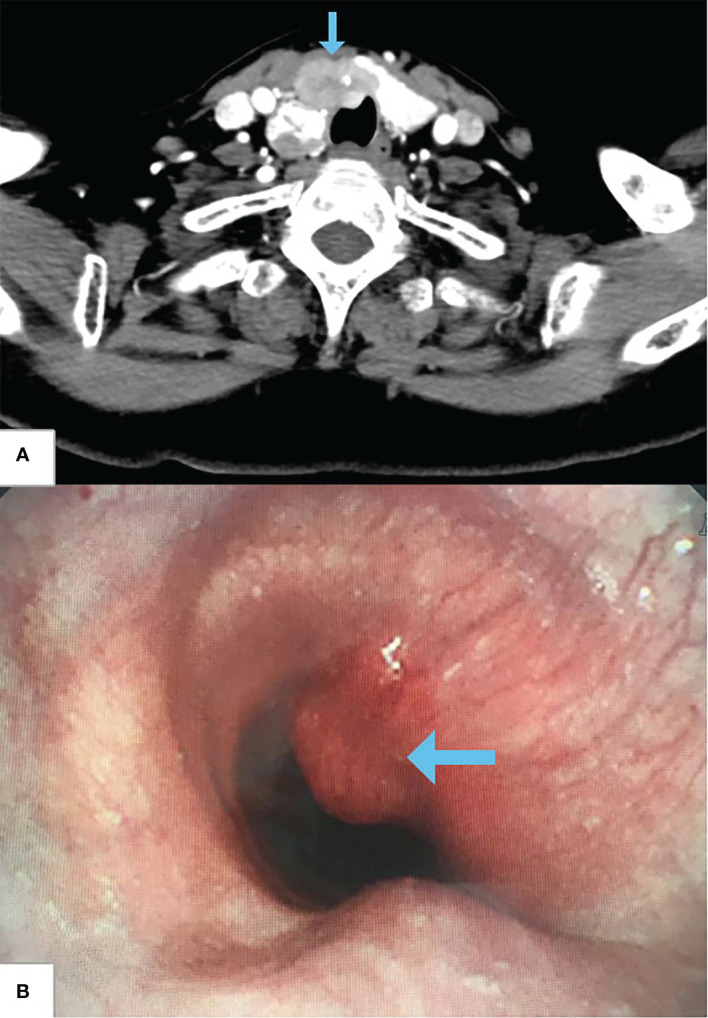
Preoperative cervical CT and fiberoptic bronchoscopy. (A) CT shows thyroid carcinoma invading trachea (arrow). (B) Fiberoptic bronchoscopy shows a protuberant tumor on the right anterior wall of the trachea, and the tracheal lumen is obviously narrow (arrow).
